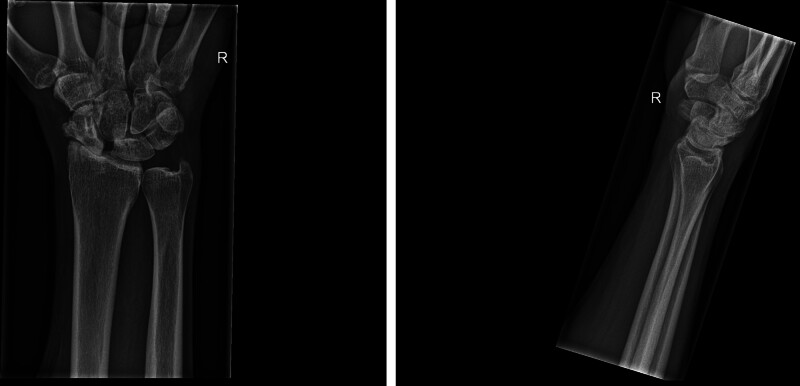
Posteroanterior and lateral wrist radiographs 6 years after index surgery, 3-LT for SLAC stage 3. There is a radiologic progression of osteoarthritis visible; clinically, the patient maintained all his activities.
